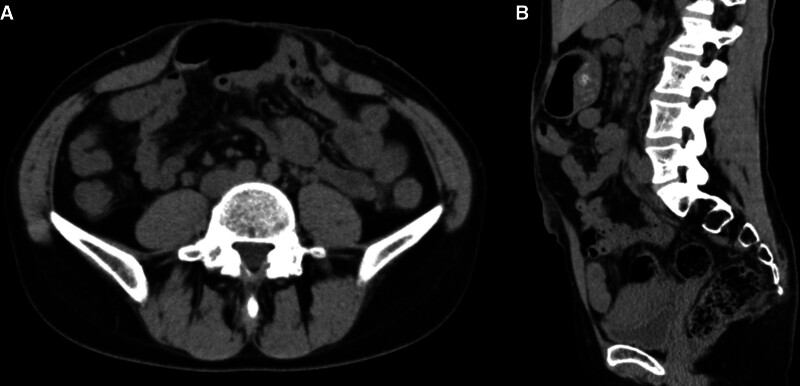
Preoperative computed tomography images of diastasis recti abdominis. Axial (A) and sagittal (B) views showing 6–7 cm of diastasis.
